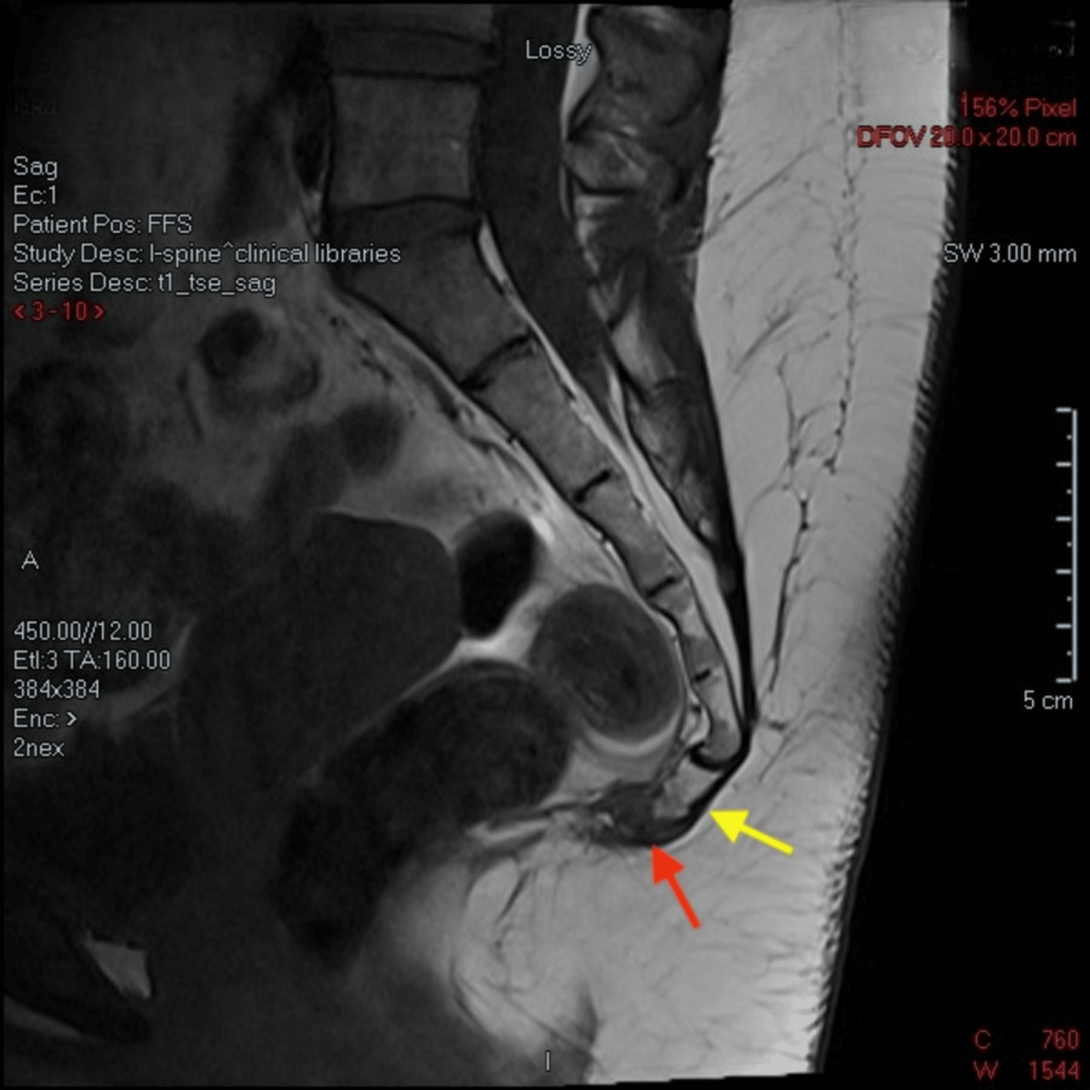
Non-contrast sagittal T1 MRI sequence Yellow arrow indicates transverse, complete, and anteriorly displaced fracture of the Cy2 segment of the coccyx, with fragment traction measuring 3 mm. Red arrow indicates a well-demarcated T1 hypointensity in the coccygeal synchondrosis, remarkable for early callous bone formation.
